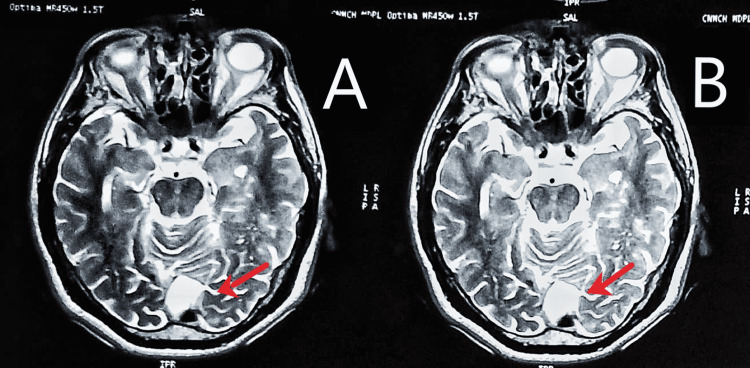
Head MRI (T2-weighted) in the axial plane
